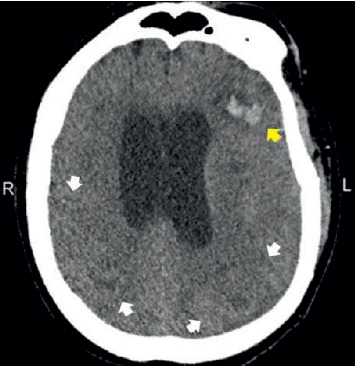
CT scan of the brain showing multiple areas of decreased attenuation in the bilateral occipital lobes and the right parietal lobe (white arrows); subacute intraparenchymal hemorrhage is present in the left frontal lobe (yellow arrow). R, right lateral; L, left lateral.
